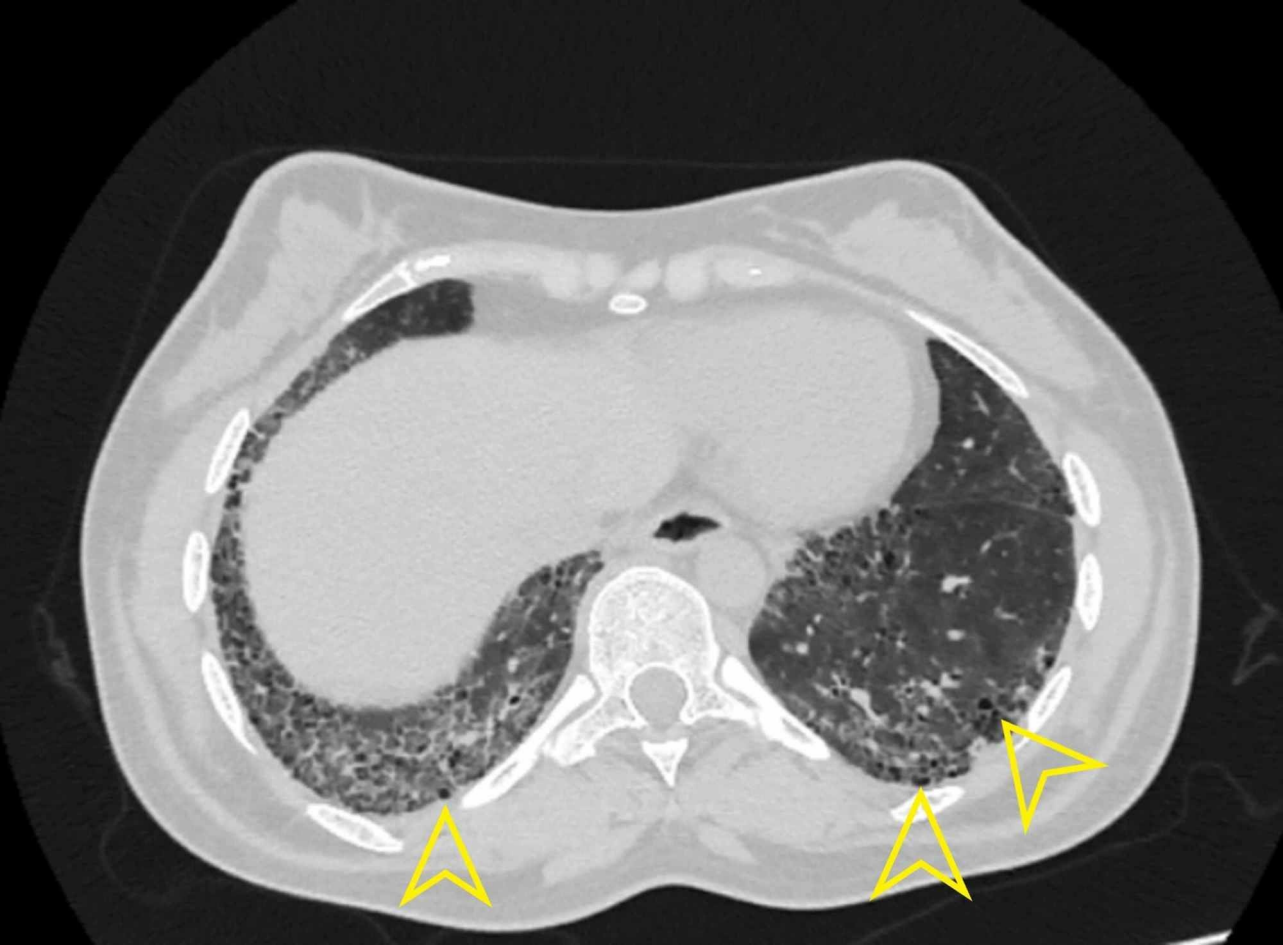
Axial section high-resolution computed tomography showing UIP Yellow arrowhead indicating honeycombing in usual interstitial pneumonia (UIP).
